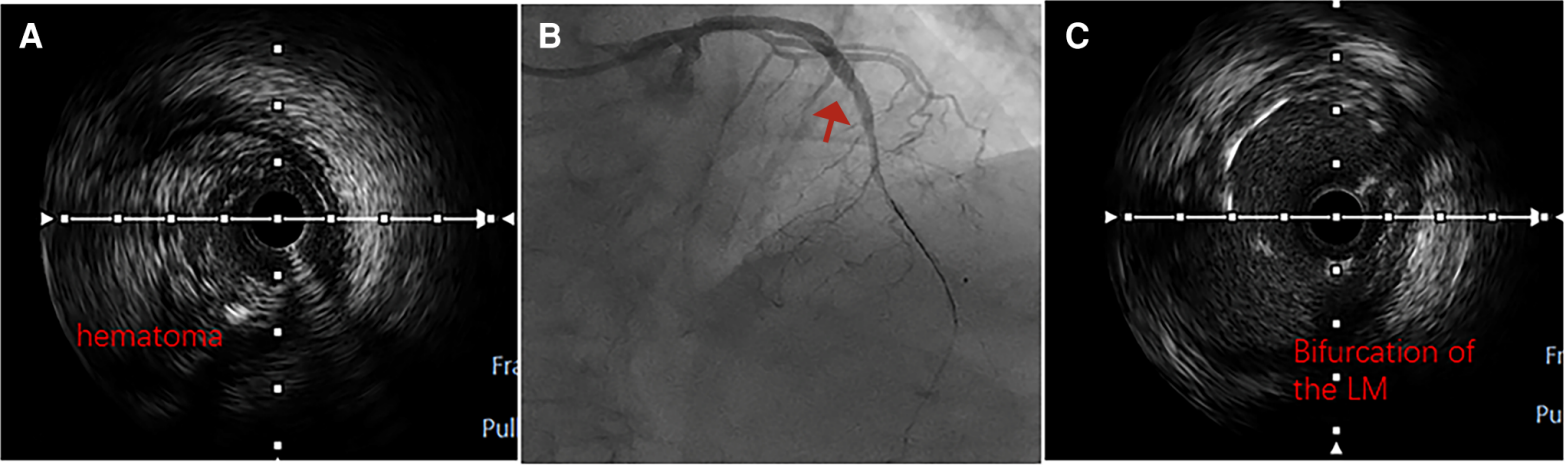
Intraoperative and postoperative intravascular ultrasound (IVUS) images, as well as coronary angiography: (A) after pre-dilation, IVUS revealed a large intramural hematoma. (B) Final angiography of the left anterior descending artery showed satisfactory apposition of the stent to the vessel wall, with no signs of dissection, and forward blood flow rated as TIMI3. (C) Intravascular ultrasound demonstrated that the stent in the left anterior descending artery extended into the left main trunk, further confirming the presence of a common origin of the left anterior descending and left circumflex arteries with the left main coronary artery measuring approximately 1 mm in length.
